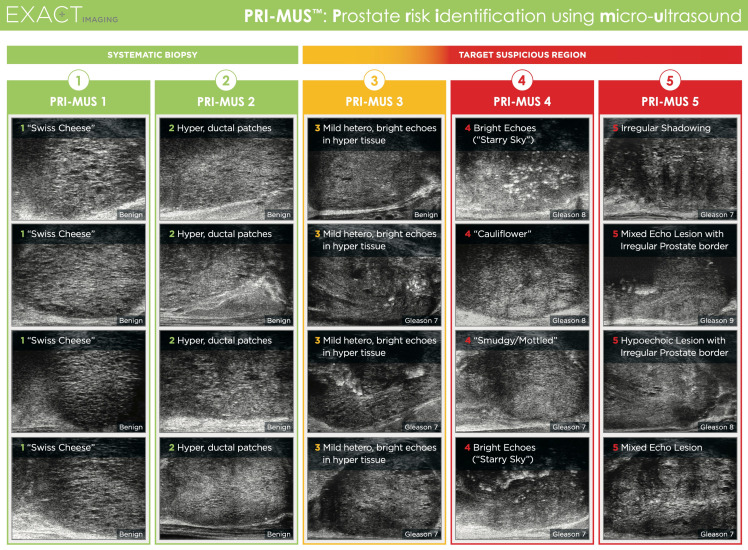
The PRI-MUS risk identification grading—The Prostate Risk Identification using Micro-UltraSound (PRI-MUS) five-point scale [24]. Image reproduced with permission from Exact Imaging, Markham, ON, Canada, 2026.
